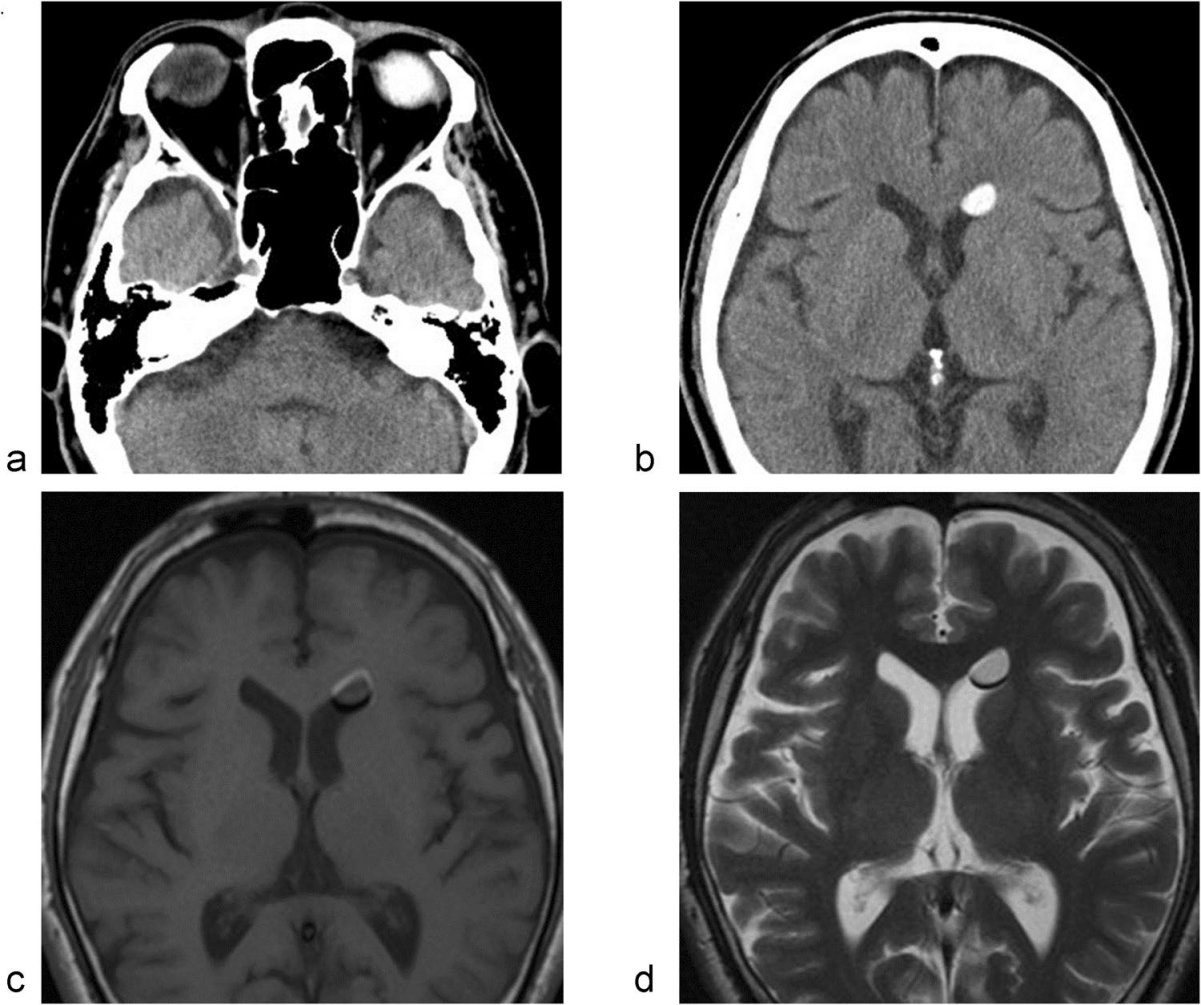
Migration of intraocular silicone oil into the brain. a, b CT, c T1-WI, d T2-WI. A 6th decade male who has silicone oil tamponade in vitreoretinal surgery for retinal detachment. After 10 years of the surgery, brain CT revealed high density material in his lateral ventricle. MRI showed chemical shift on both T1 and T2-WI, indicating silicone oil transport into the brain. The migration of the silicone oil is speculated to have taken place via the subarachnoidal space around the optic nerve
